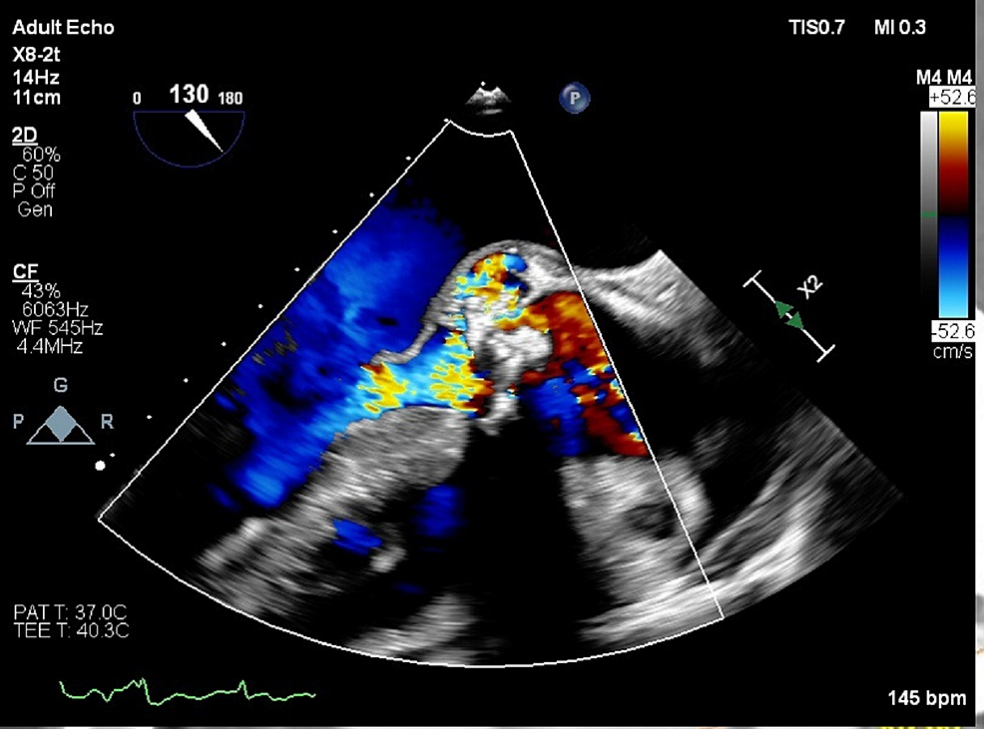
Pre-operative transesophageal echocardiogram. Mid-esophageal aortic valve long-axis view with color Doppler showing severe aortic regurgitation.
